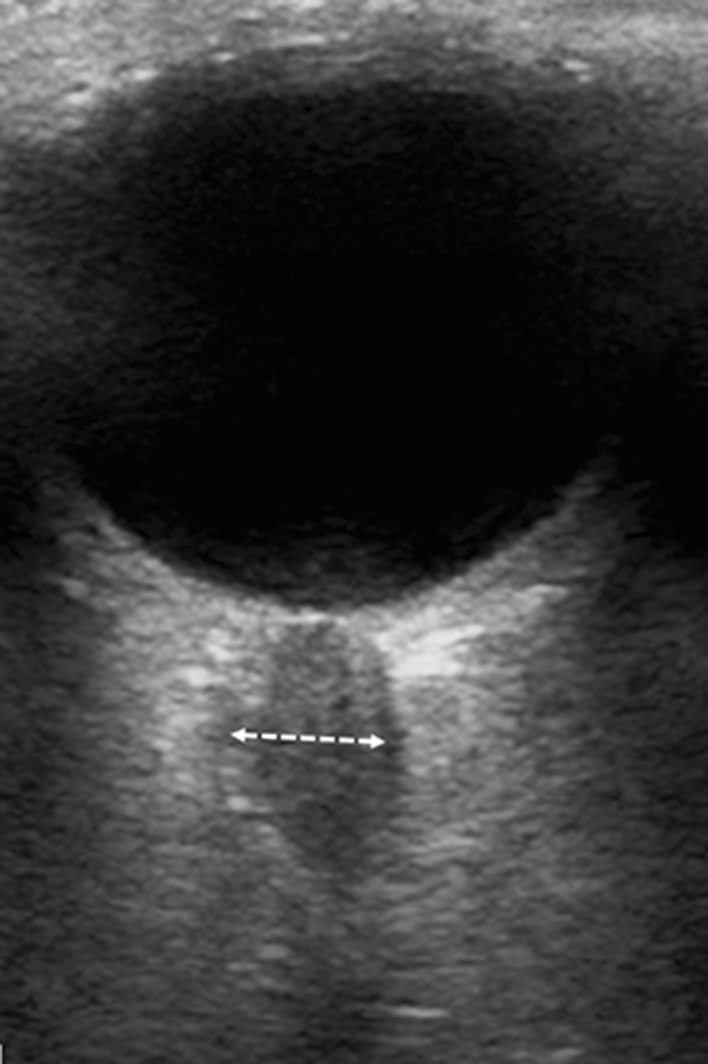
An ultrasonographic image of optic nerve sheath diameter (ONSD) measurement (marked by a dotted arrow)
